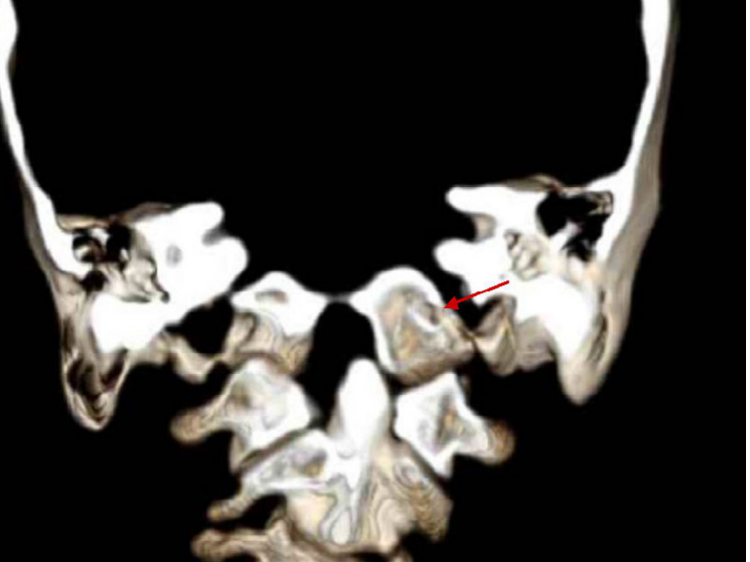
Coronal computed tomographic reconstructions demonstrating dislocation at C1-C2 and Subluxation of the occipito-atlas junction. Note C2 in the coronal position with the anterior arch of C1 overlying the left facet. Sphenoide bone, anterior part of occipital foramen (arrow).
